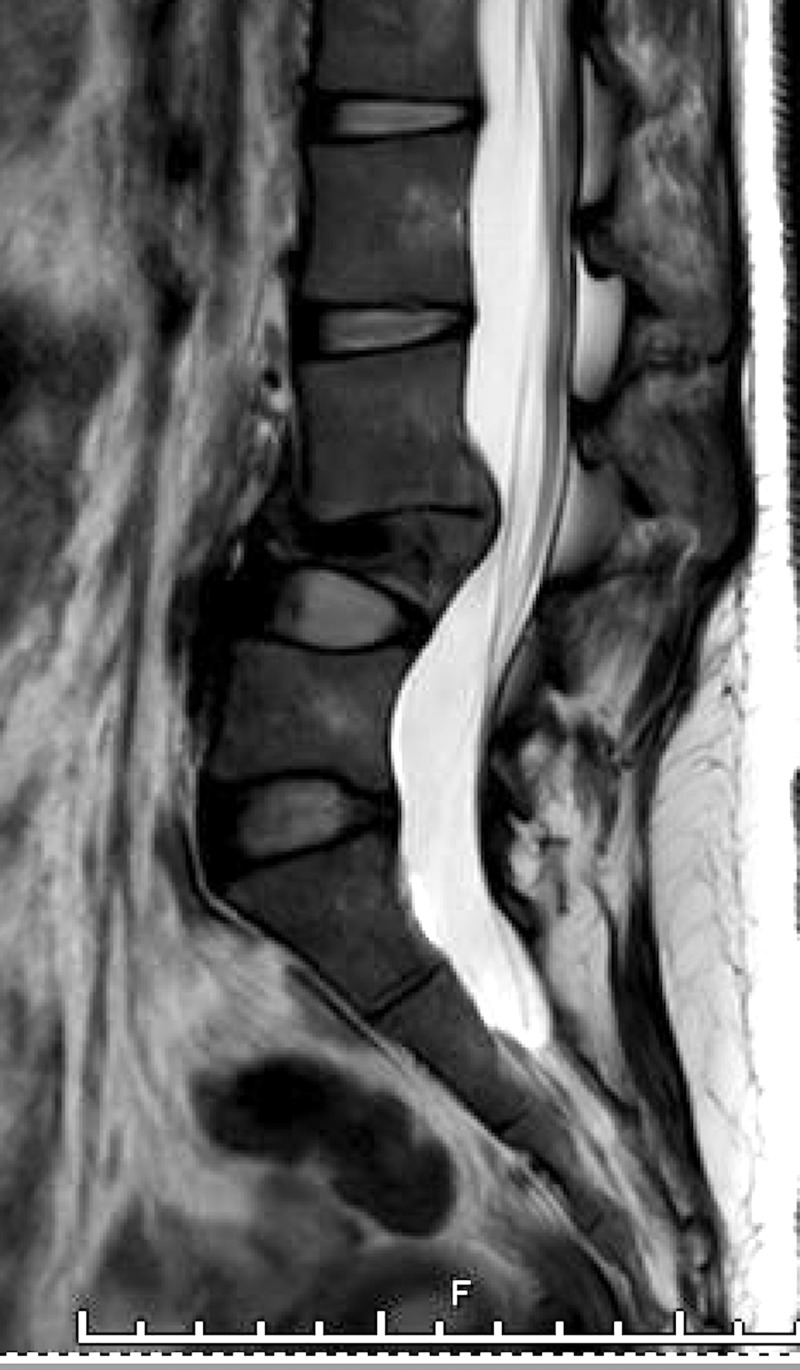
Lumbar MRI with new deformity Sagittal T2-weighted MRI showing increased compression and anterior wedging of L4 vertebral body with subsequent kyphosis.
